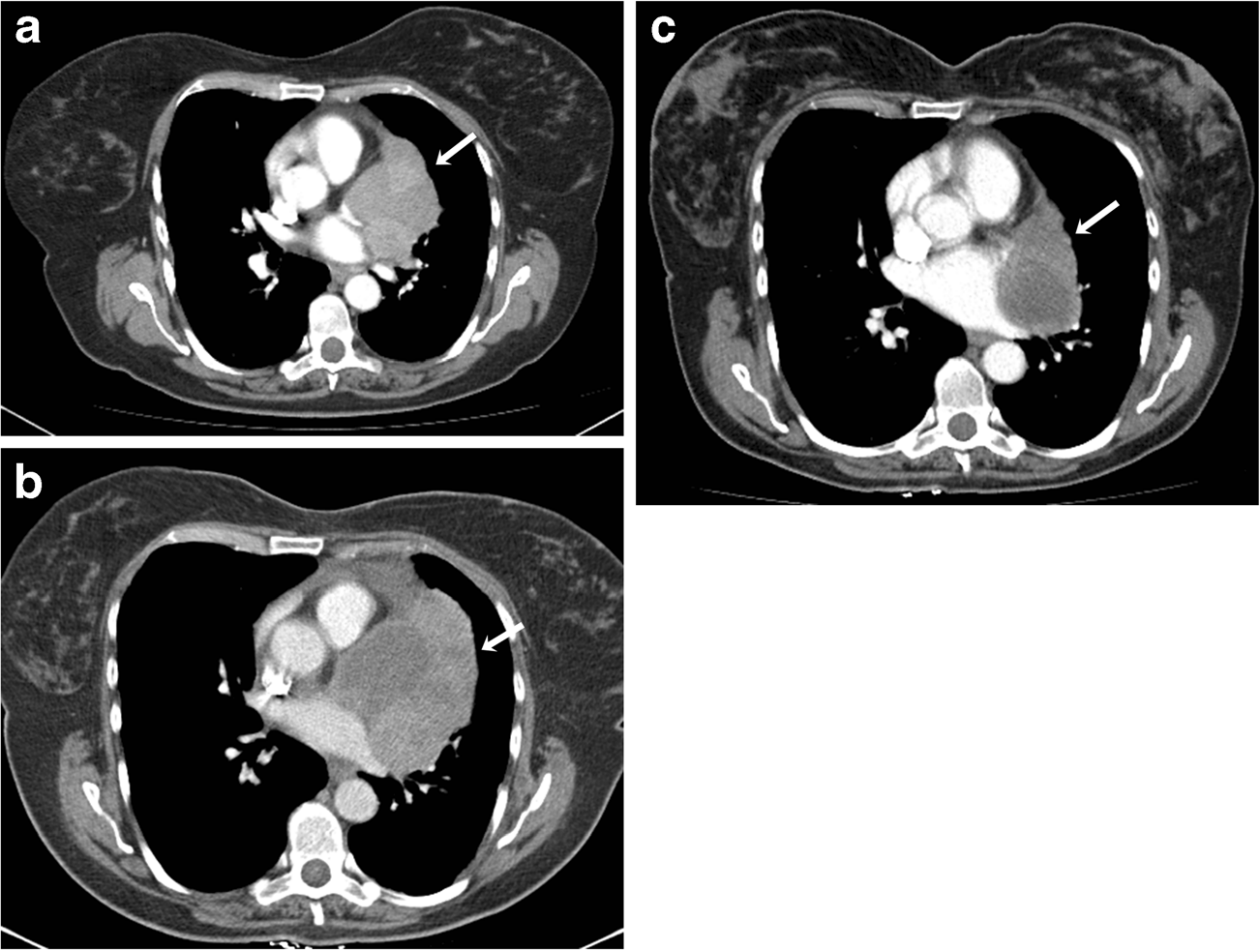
A 57-year-old female with metastatic melanoma from anorectal primary on treatment with ipilimumab. a Axial contrast-enhanced CT image before the start of chemotherapy shows a large heterogeneous paracardiac mediastinal mass (arrow). b Axial contrast-enhanced CT image 1 month after the start of treatment shows an increase in the size of the mass, however with a decrease in enhancement (arrow). c Axial contrast-enhanced CT image 1 month after completion of treatment shows a decrease in the size and enhancement of the mass (arrow)
